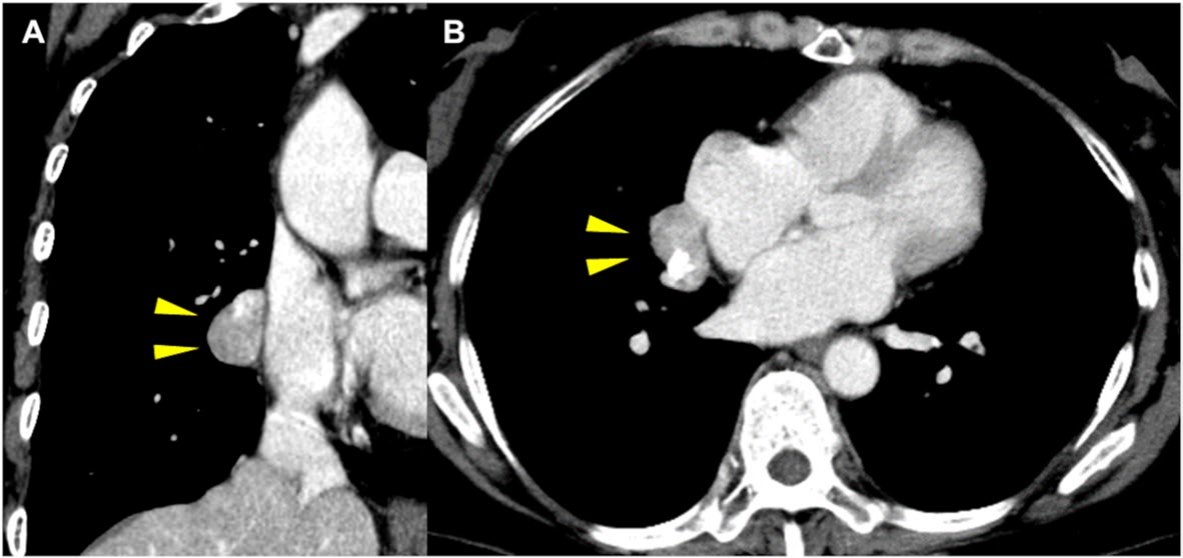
A contrast-enhanced CT image. A, B A contrast-enhanced CT image of the chest highlights a 3 cm heterogeneous mass with calcification in the mediastinum. Yellow triangles indicate the tumor
